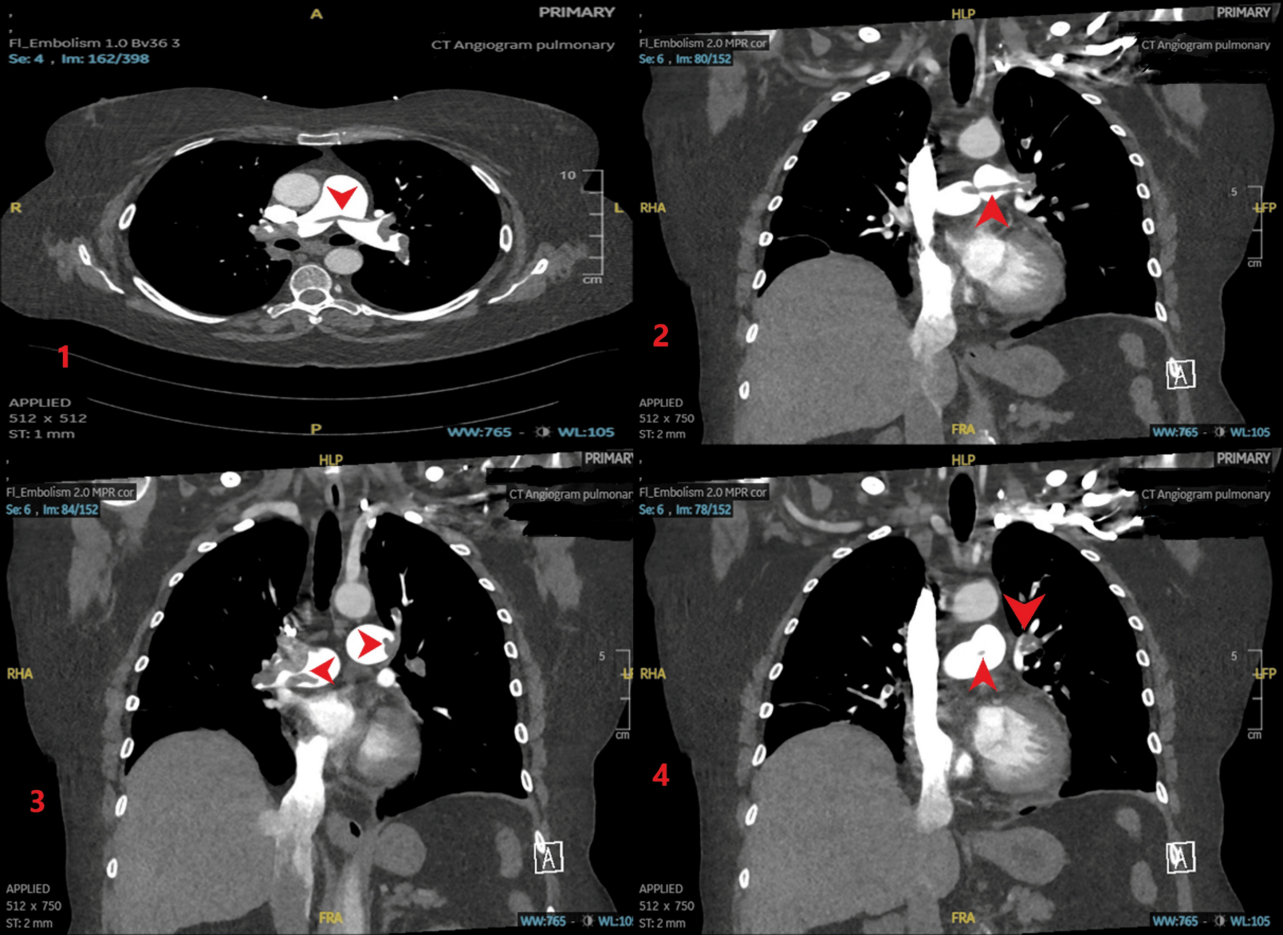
The CTPA showing saddle and bilateral segmental PE, and evidence of pulmonary hypertension and right heart strain. CTPA: Computed tomography pulmonary angiography, PE: Pulmonary embolism
